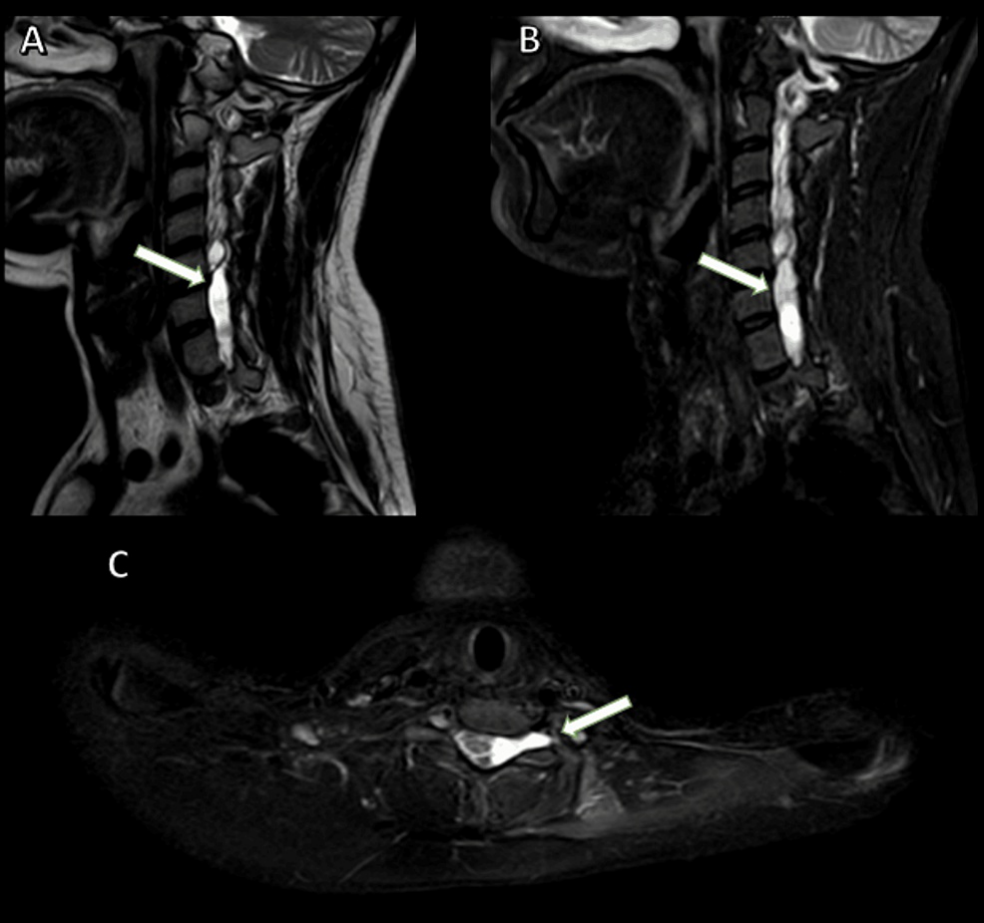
MRI-brachial plexus (A) T2 left parasagittal section, (B) STIR left parasagittal section, (C) STIR axial section of case 2 MRI: Magnetic resonance imaging; STIR: short tau inversion recovery; CSF: cerebrospinal fluid MRI of the brachial plexus (A) T2-weighted left parasagittal view, (B) STIR left parasagittal view showing extradural collections that resemble the CSF within the spinal canal, located laterally on the left side at the levels of the C4-C5, C5-C6, and C6-C7 vertebrae. These collections do not contain central neural elements and are bordered by margins that transition from smooth to lobulated, creating a significant mass effect and displacing the cervical cord laterally to the right. (C) STIR axial view, showing CSF-filled structure extending into the left neural foramen at the levels of the C6-C7 vertebrae, indicated by a large thick white arrow.
